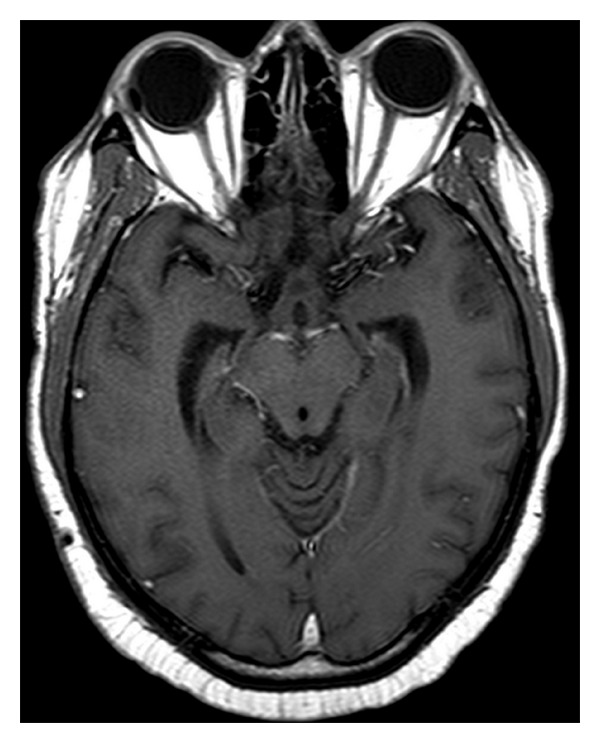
Cerebral T1-weighted MRI posttreatment: the carcinomatosis disappeared.
